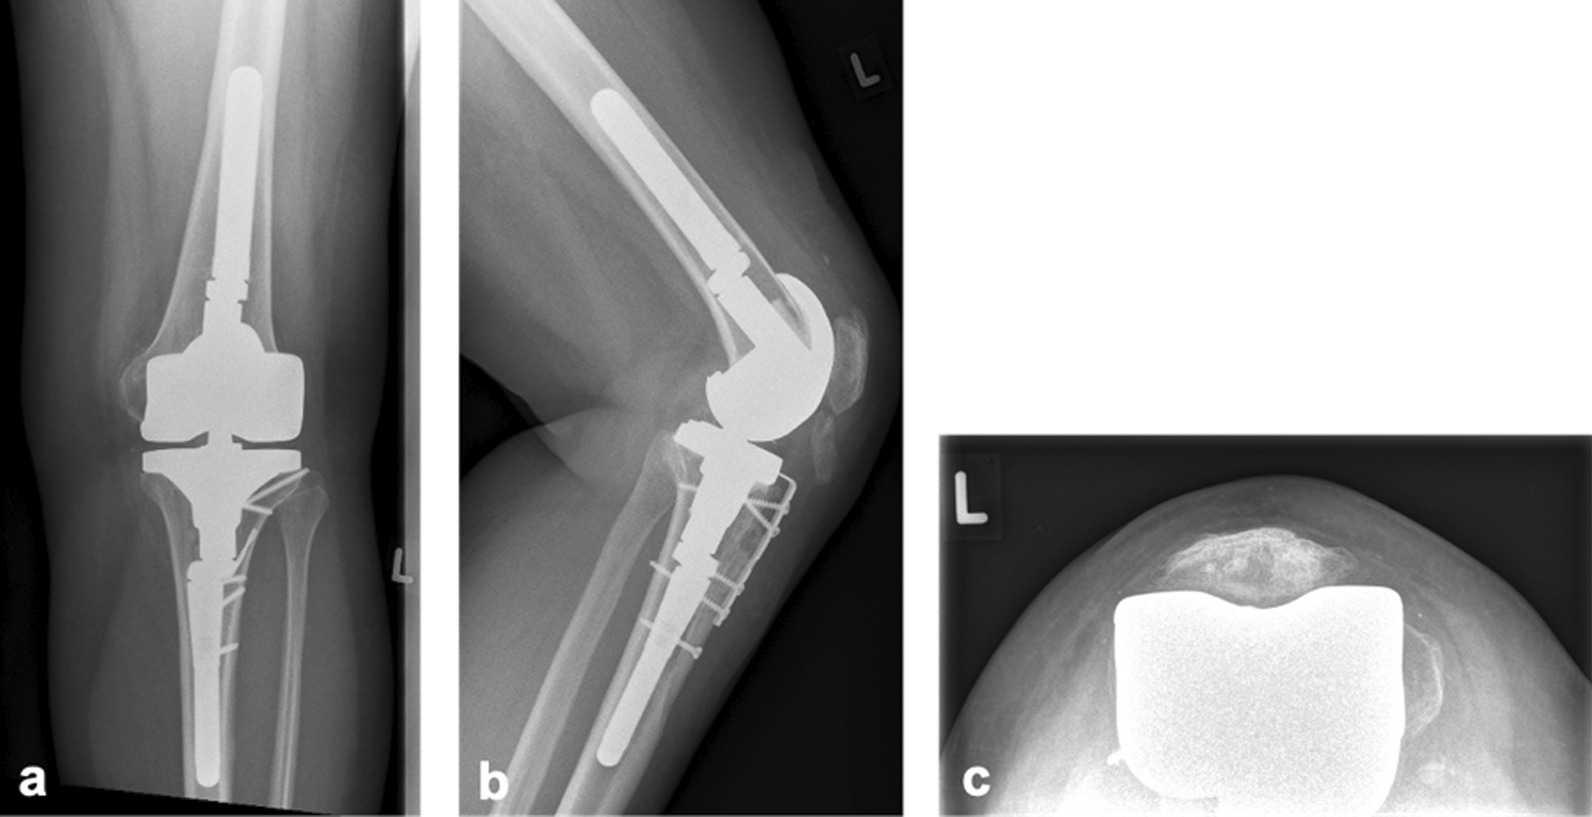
Preoperative x-ray shows dislocated tibial tubercle, one-third plate, rotation hinge prosthesis without loosening signs and regular patella tracking. a Frontal view. b Sagittal view shows dislocated tibial tubercle. c Axial view shows regular patella tracking
